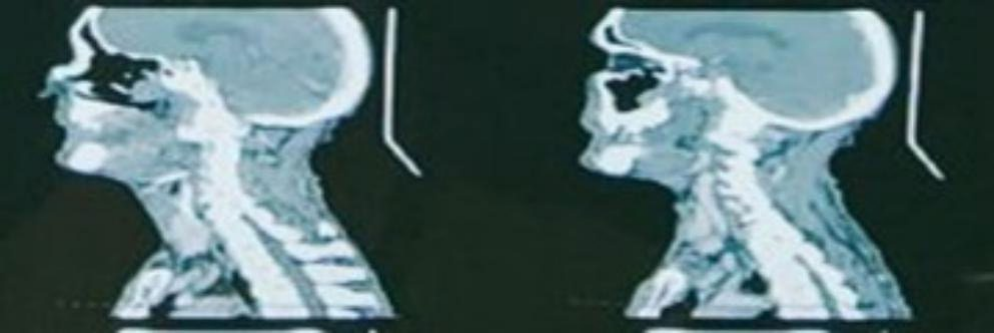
CT imaging showing extension of lesion into left pterygopalatine fossa with extension into left maxillary sinus eroding its postero-lateral wall
